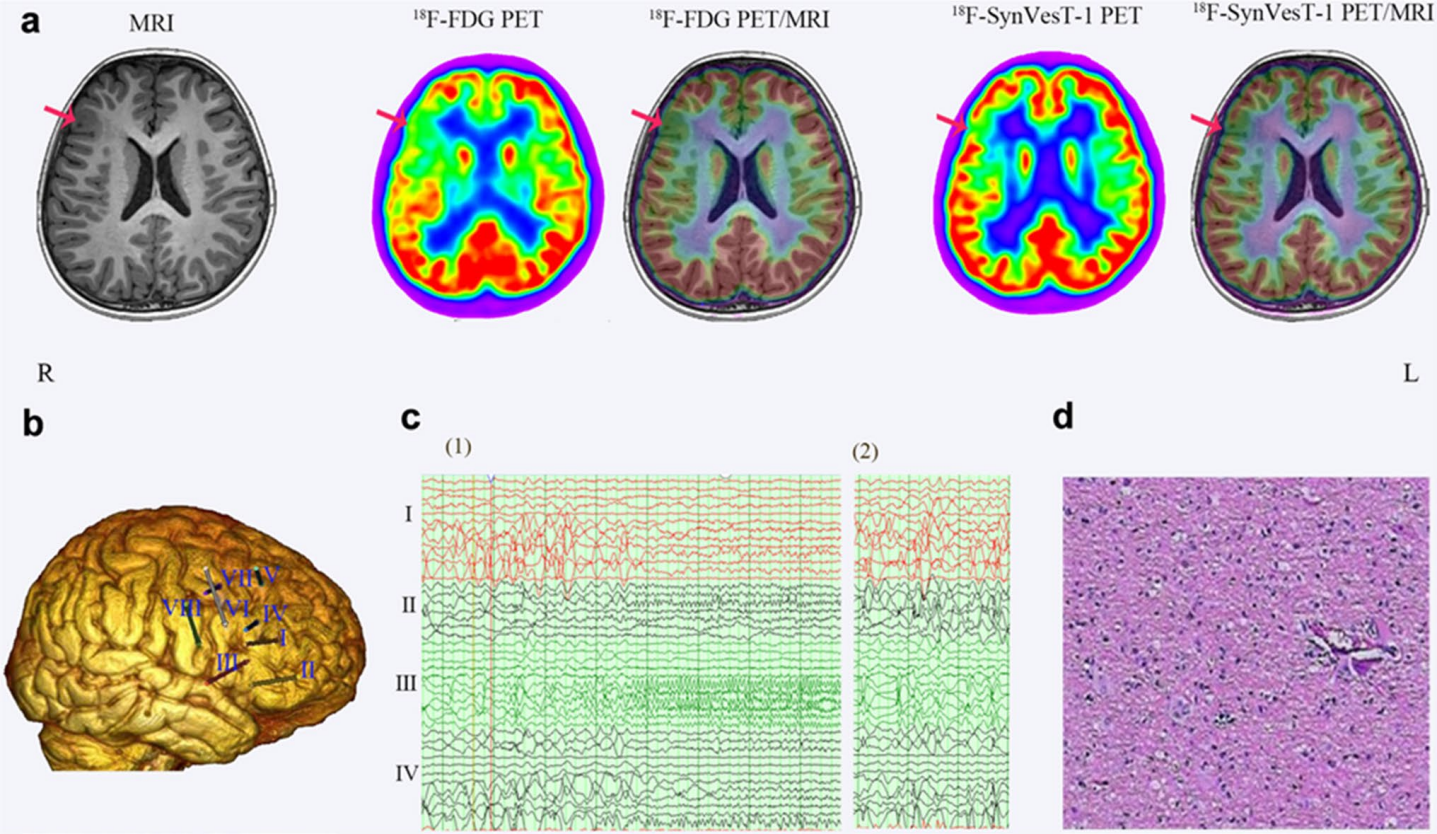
MRI, ^18^F-FDG, and ^18^F-SynVesT-1 PET/MRI images used for localization of the FCD II lesion in patient 8. a MRI image showed the thickening of the right inferior frontal gyrus; ^18^F-FDG PET and ^18^F-FDG PET/MRI images showed hypometabolism throughout the right inferior frontal gyrus and surrounding areas; ^18^F-SynVesT-1 PET and ^18^F-SynVesT-1 PET/MRI showed a more restricted area of low uptake in the frontalis inferior region (red arrows). b Overall electrode placement view. c Ictal (1) and postictal (2) SEEG recordings showed that the abnormal discharge area originated from the inferior frontal gyrus (II and III). d Postoperative pathology showed FCD IIb
